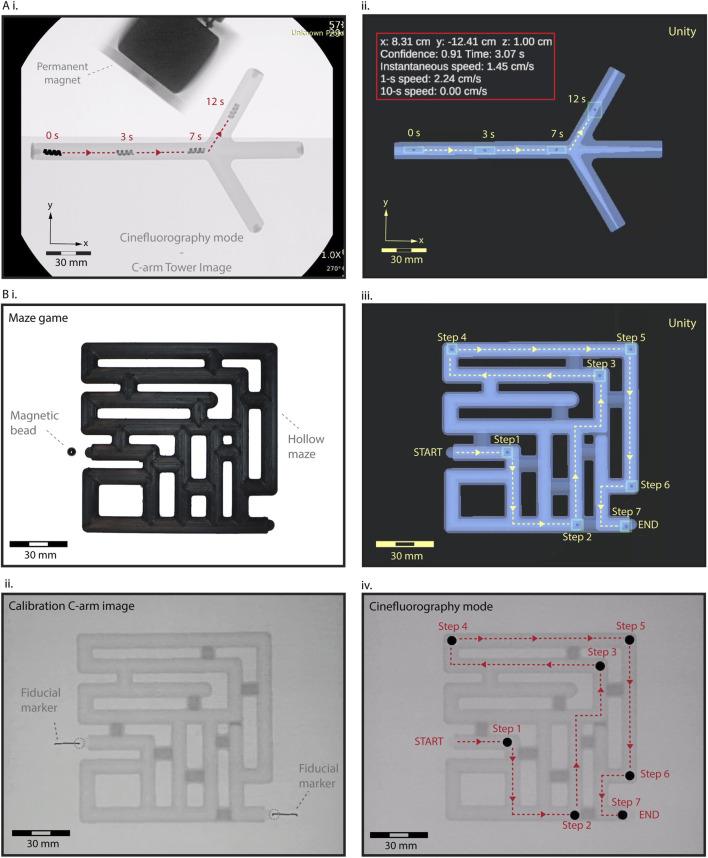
Fluoroscopic-guided real-time localization and telerobotic steering control in the digital twin interface. (A) (i) A real-time validation and control HMS steering. As the magnet and a segment of the robot arm traverse the imaging field, the relative contrast between the HMS and background visibility fluctuates. However, the detection algorithm is not affected by these fluctuations, maintaining the tracking robustness (ii) Spatiotemporally precise steering control of HMS from the virtual feedback within the digital twin. (B) A maze-solving game to demonstrate tracking and steering capabilities using the virtual feedback. (i) Initial RGB camera image showing a closed maze setup with a NdFeB magnet bead positioned at the start. (ii) The first X-ray image captures the maze with fiducial markers, used to calibrate and align the virtual and real-world coordinates before initiating telerobotic navigation. (iii) Sequential tracking and navigation of the bead through the virtual maze twin, from START to END, using stepwise control of the robot arm for remote magnetic actuation. (iv) Final validation of the magnetic bead’s localization within the maze under the C-arm, confirming the accuracy of the virtual navigation system.
